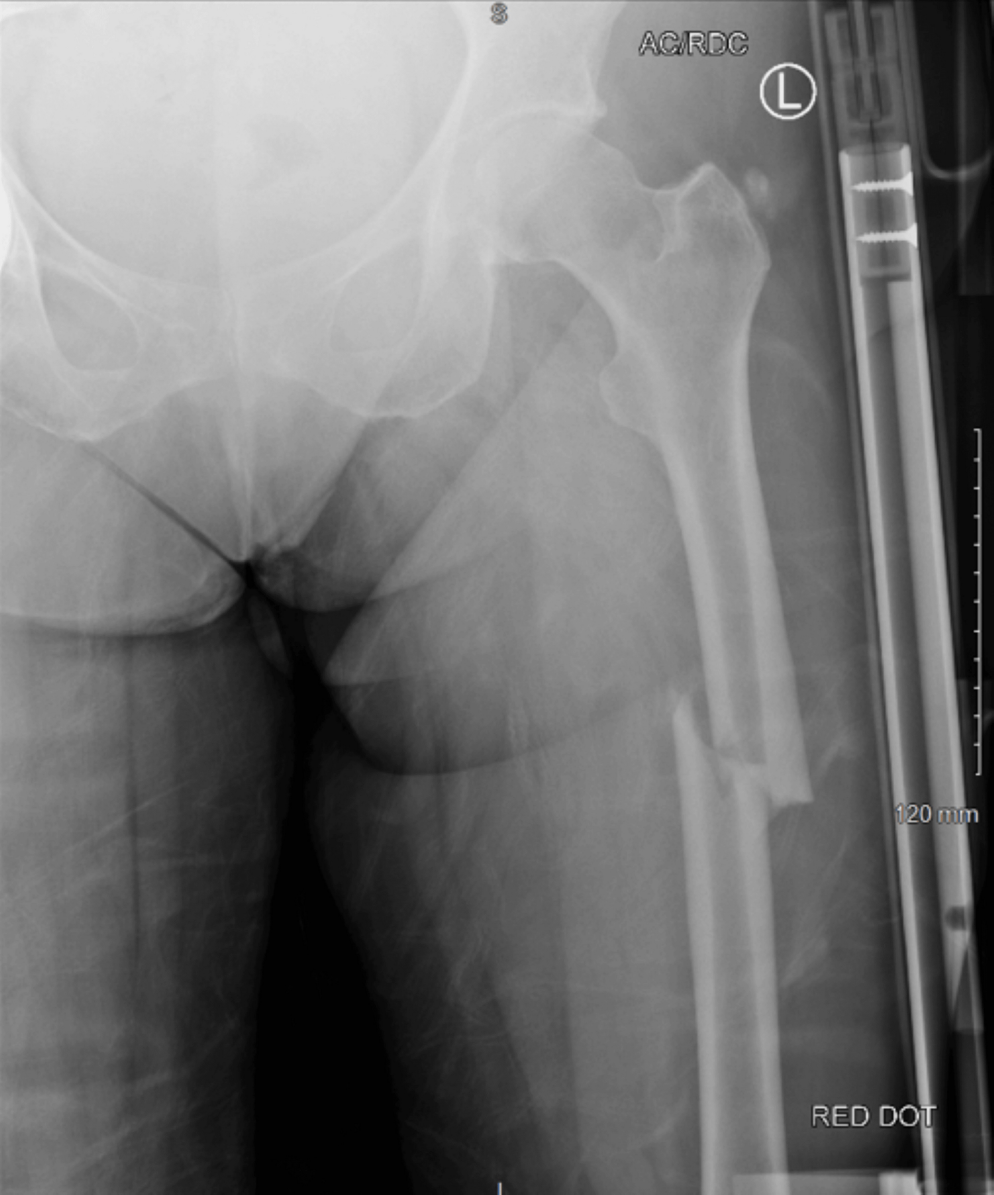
Anteroposterior X-ray of the left femur after the accident from the hospital providing primary care
